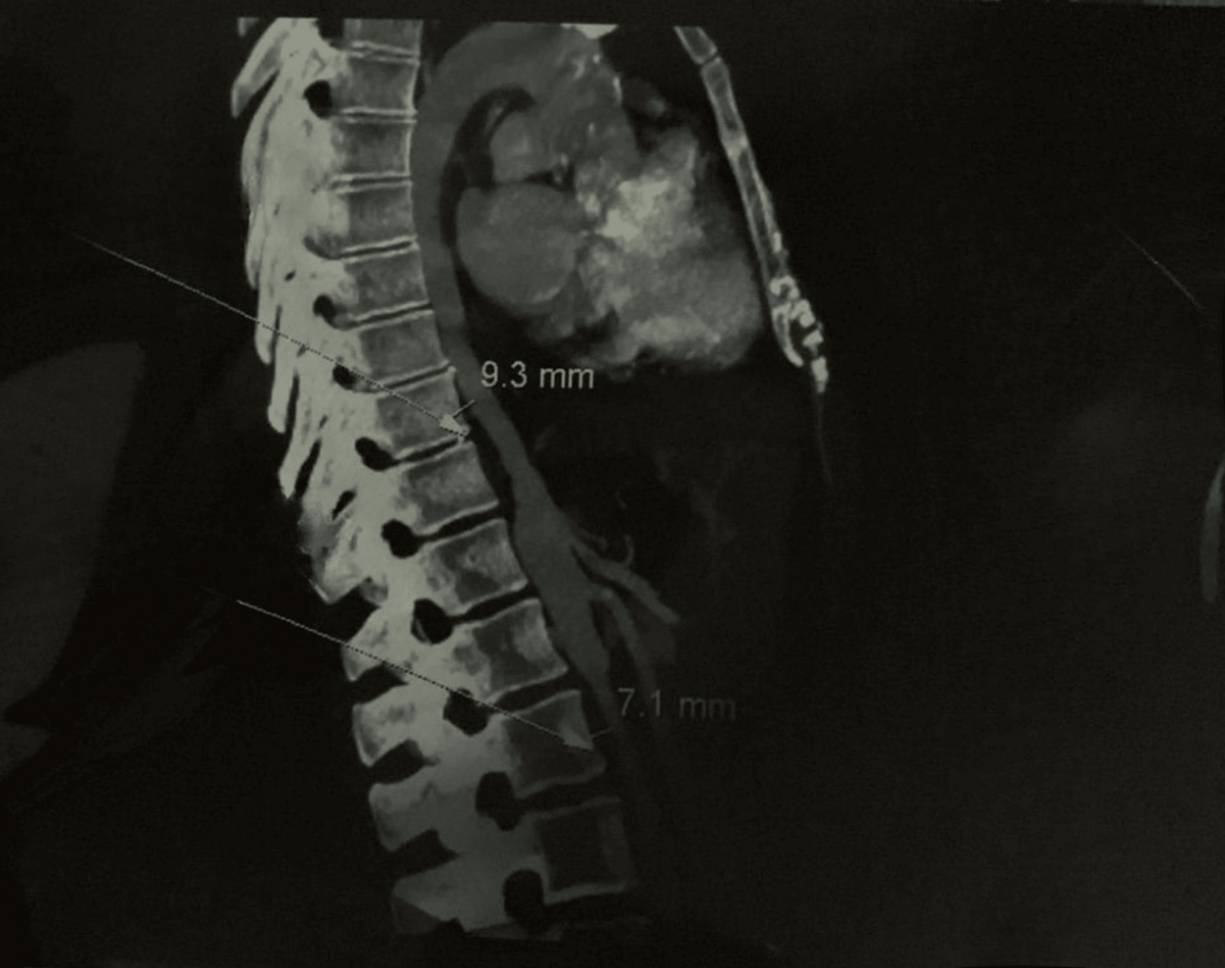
Thoracoabdominal aorta: segmental wall thickening. Axial CT angiography reveals mural thickening of the thoracoabdominal aorta, measuring 9.3 mm in the descending thoracic segment and 7.1 mm in the infrarenal abdominal segment. Arrows indicate the respective sites of measurement.
